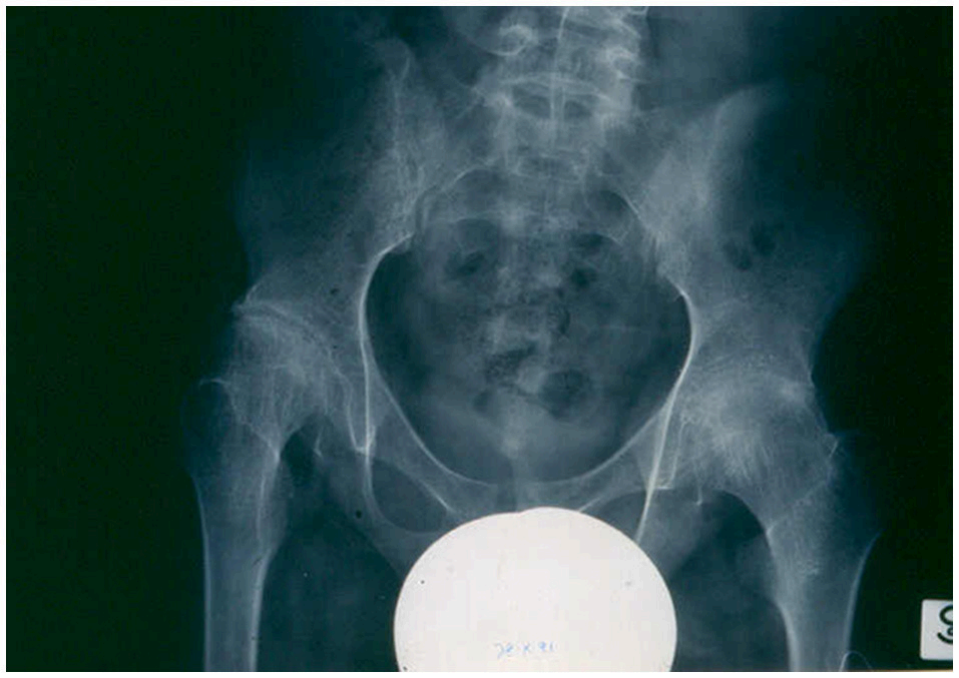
X-ray showing advanced destructive changes in the hips of a systemic JIA patient.
